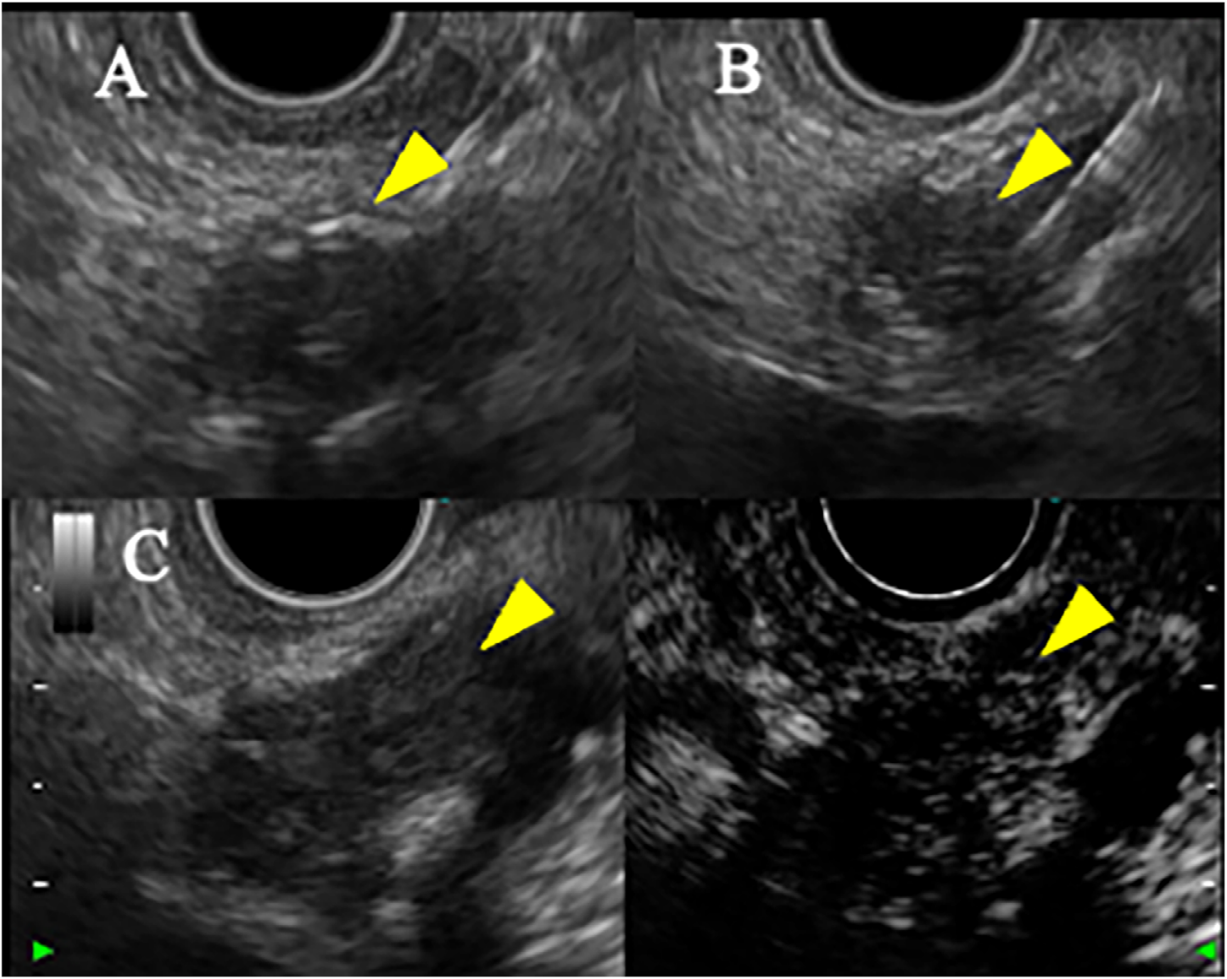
Findings on endoscopic ultrasound. (A) A 20‐mm hypoechoic mass with relatively clear borders was seen in the pancreatic body. (B) Endoscopic ultrasound‐guided fine‐needle biopsy was performed on the mass in the pancreatic body via the stomach. (C) Contrast‐enhanced harmonic endoscopic ultrasound showed a slight enhancement of the mass in the pancreatic body from an early stage.
